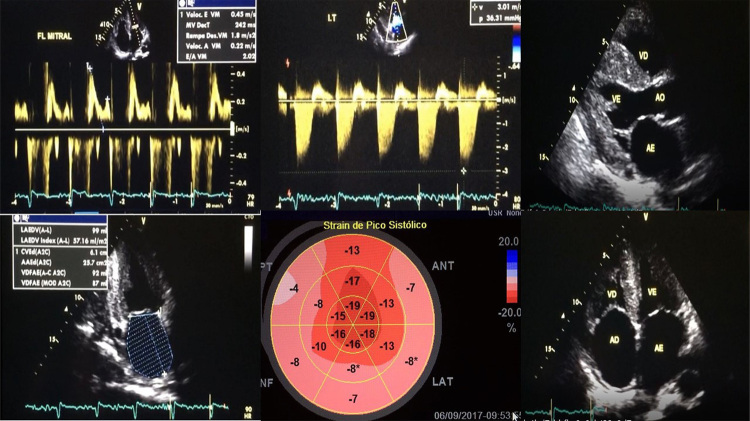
Ecocardiograma transtorácico mostrando padrão do Doppler mitral do tipo pseudonormal (disfunção diastólica tipo II), pico de velocidade da insuficiência tricúspide > 2,8 m/s e aumento biatrial com volume indexado do átrio esquerdo de 87 ml/m2. Cortes longitudinal paraesternal e apical 4 câmaras mostrando aumento do brilho miocárdico, espessamento do septo interatrial e das valvas e HVE. Strain global longitudinal com padrão típico de amiloidose - "relativa preservação das regiões apicais" e redução do strain longitudinal nos segmentos basal e médio do miocárdio.
